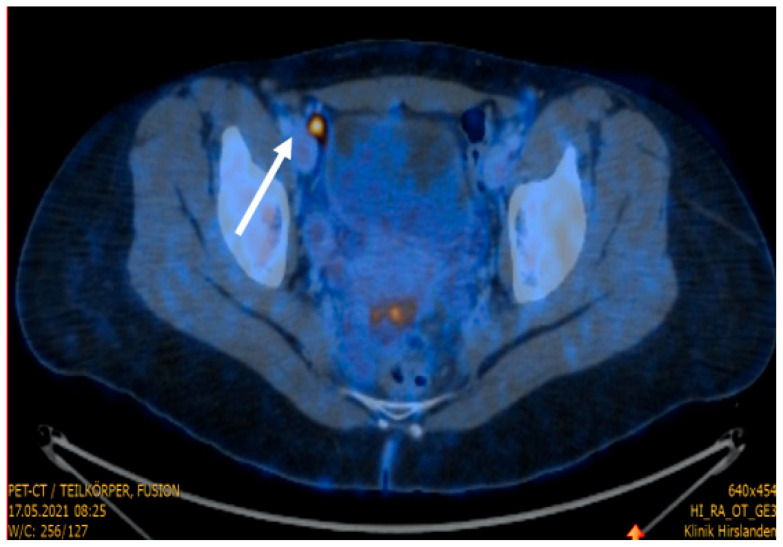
PET/CT showing a metabolically active lymph node. A centrally hypodense, metabolically active lymph node in the right external iliac region (SUVmax 9.5), measuring 12 mm in transverse diameter (white arrow).
